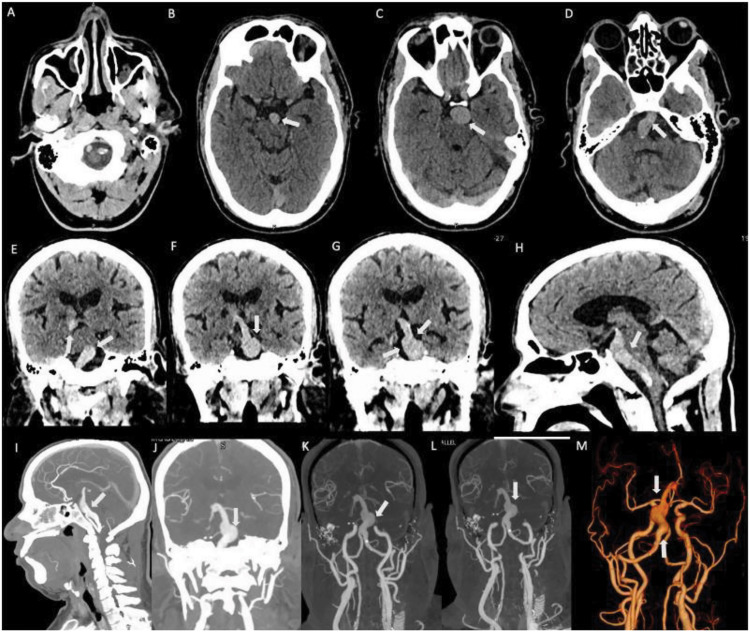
Computed tomography (CT) scan of the head and CT angiogram of the head and neck. (A–D) Non-contrast head CT scan showing the saccular dilatation of the basilar artery trunk. (E–M) CT angiogram of the head with intravenous (IV) contrast showing the aneurysmal dilatation of the basilar artery trunk.
